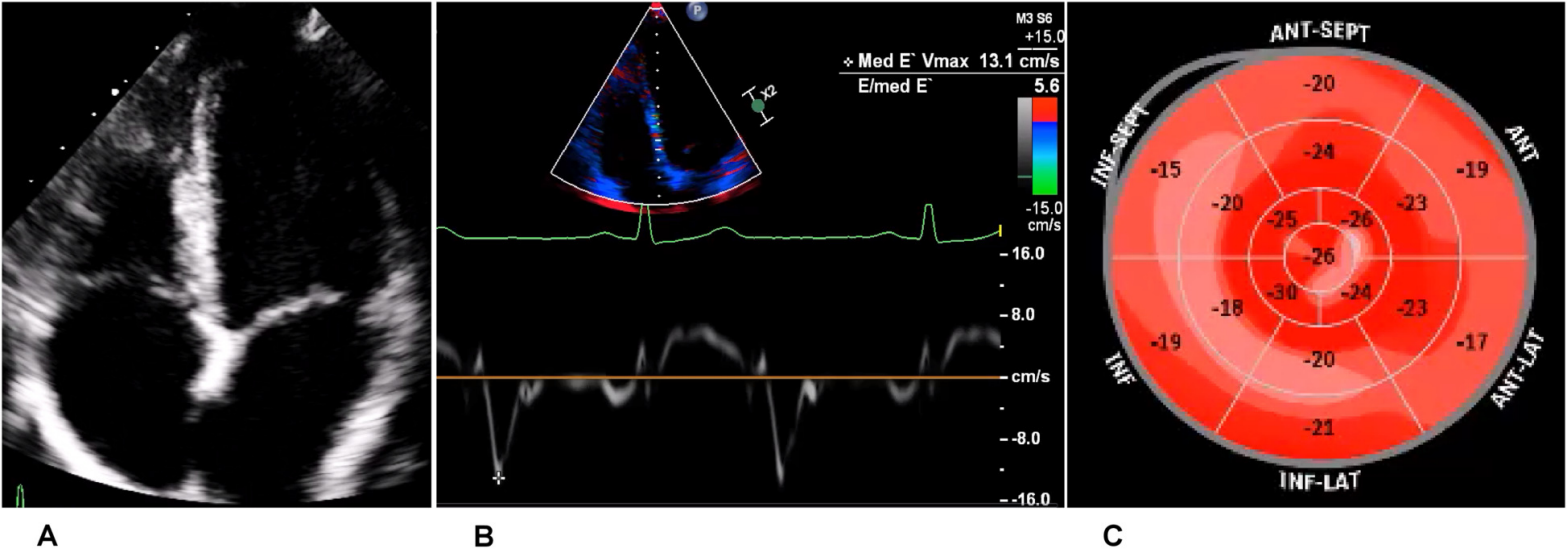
Echocardiography of the recreational athlete (ice skating). (A) Four-chamber view on transthoracic echocardiography showing no signs of structural heart disease. (B) Medial tissue Doppler imaging demonstrating velocity within the normal range. (C) Bullseye display of the distribution of longitudinal strain, with more positive values depicting worse strain. This was demonstrated in the inferior, inferolateral, and inferoseptal regions, corresponding to the LGE on magnetic resonance imaging.
